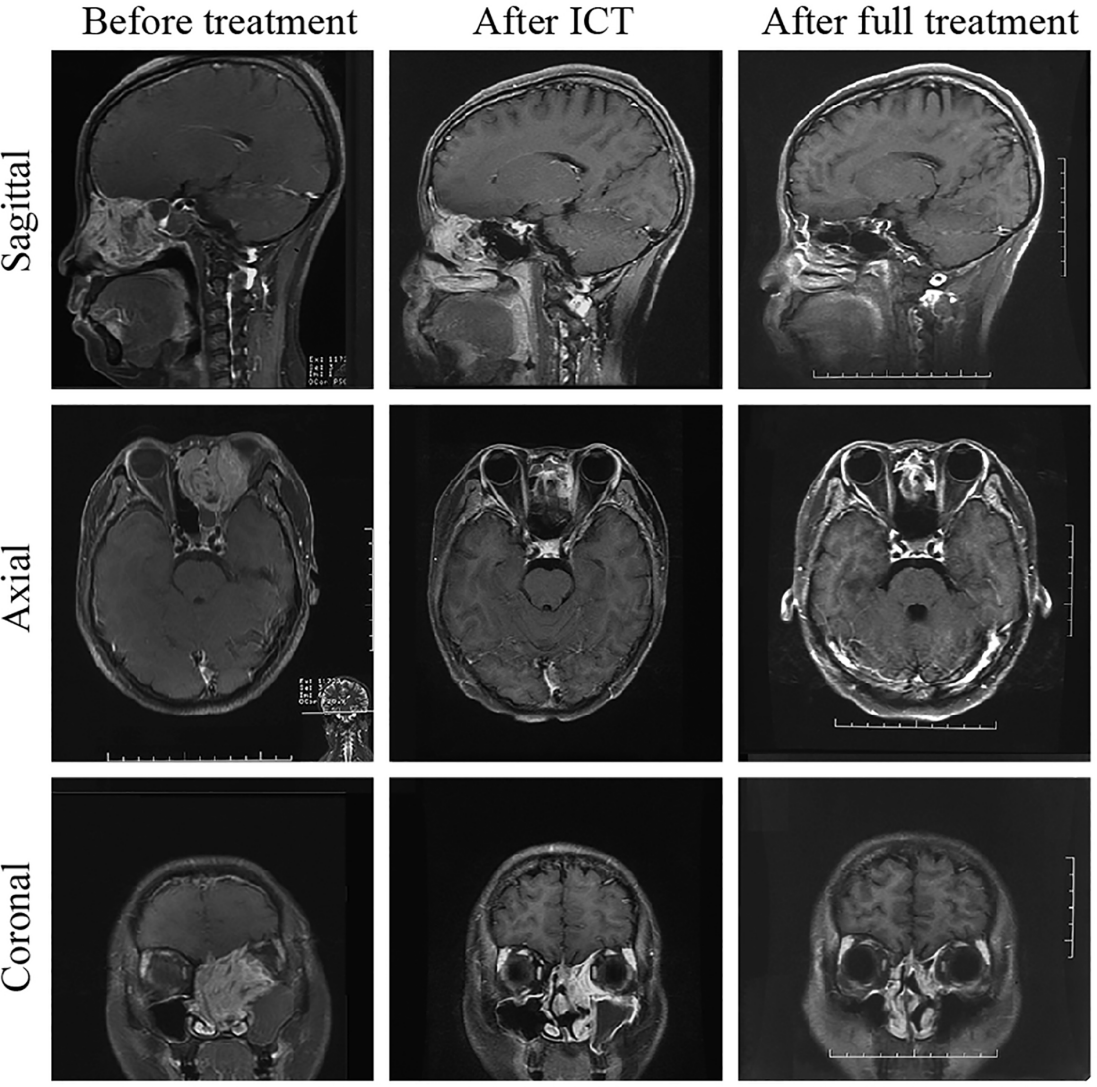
Comparison of MRI before and after treatment of one patient with rhabdomyosarcoma. The lesion size was significantly reduced after ICT; 1 month after treatment, the tumor had completely subsided. The patient was followed up for 52 months and remained free of disease.
